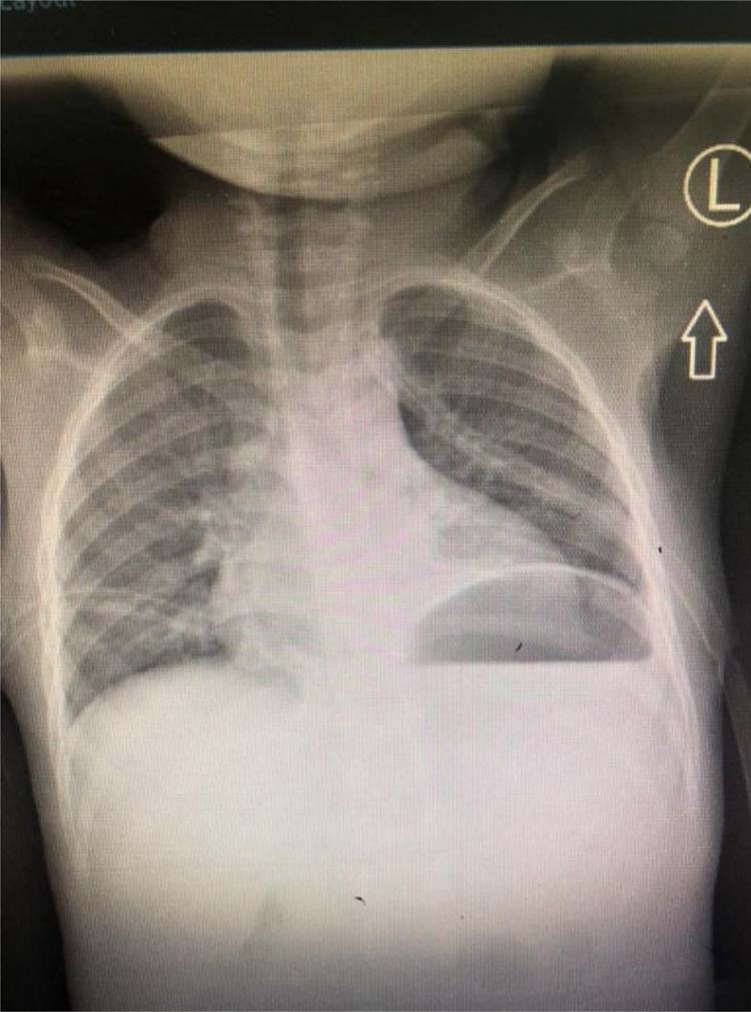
Control Chest X-ray showing resolution of the chylothorax with thoracostomy tubes *in situ*.
